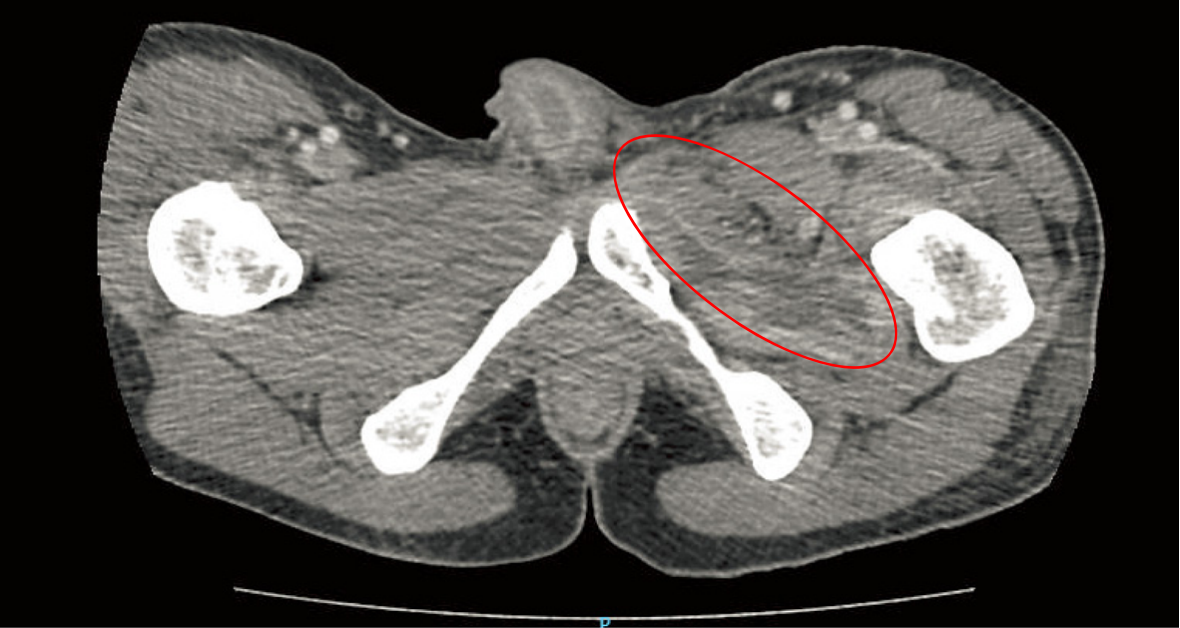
CT Abdomen and Pelvis cut demonstrating the collection inferiorly extends to a soft tissue defect over the medial thigh, with the largest pocket at the adductor muscles measures approximately 8.8 × 2.7 cm
